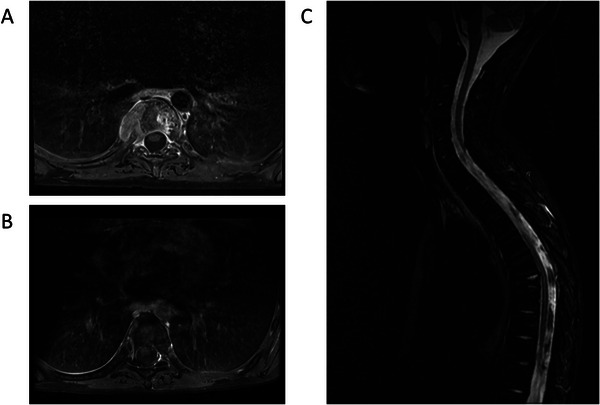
Selumetinib response pre‐HSCT transplant and persistence of long‐term remission. (A) Axial T1FS gadolinium MRI sequences showing reduction of the extra‐osseous component. (B) No residual paravertebral mass at T7 visualized in axial T1FS gadolinium MRI sequence. (C) Regression of the sagittal STIR hypersignal of the T7 vertebra with no sign of epiduritis or spinal cord abnormalities but persistent vertebral compression at T7.
